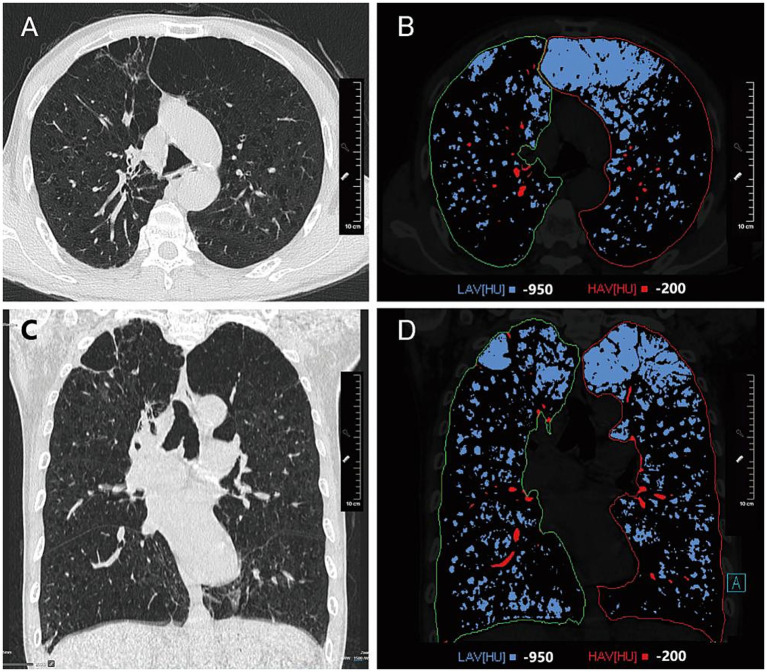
CT quantification of emphysema using the %LAA_−950_ index. The left column shows lung-window CT images in the axial (A) and coronal (C) planes. The right column shows 3D-reconstructed CT images in the axial (B) and coronal (D) planes. The blue areas represent lung regions with low attenuation areas below −950 HU (indicative of emphysema), and the red areas represent high attenuation areas above −200 HU. In this subject, the %LAA_−950_ was 36%. %LAA_−950_, the ratio of low attenuation areas below −950 HU to total lung volume measured by CT scan.
